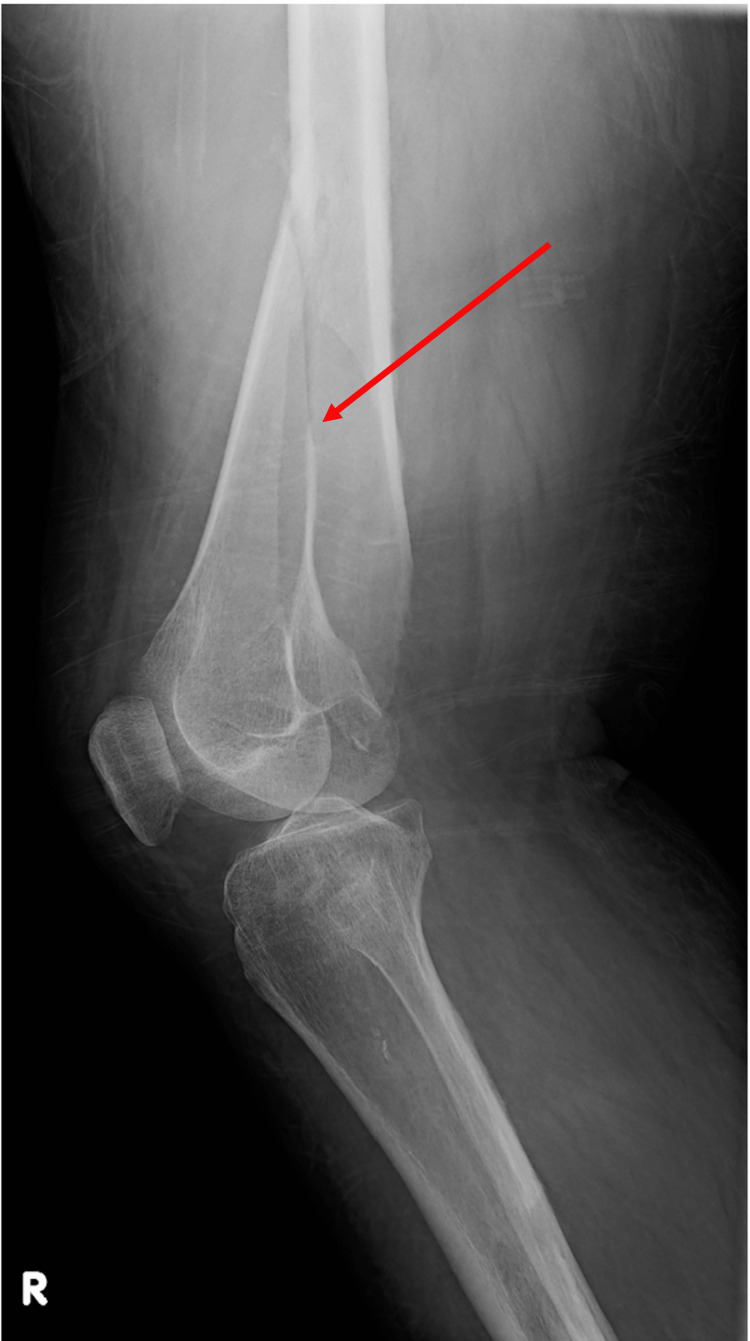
Lateral radiograph of the right knee demonstrating an obliquely oriented, displaced, mildly angulated acute fracture (red arrow) of the right distal femoral metadiaphysis with overriding of the fracture fragments.
